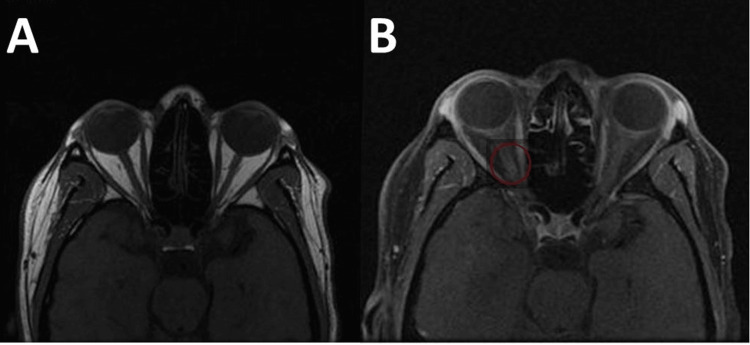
Axial T1 MRI of the orbits at the level of the optic nerve: (A) precontrast, unremarkable; (B) postcontrast, asymmetric hyperenhancement of the right optic nerve compared with the left side (circled). MRI, magnetic resonance imaging
